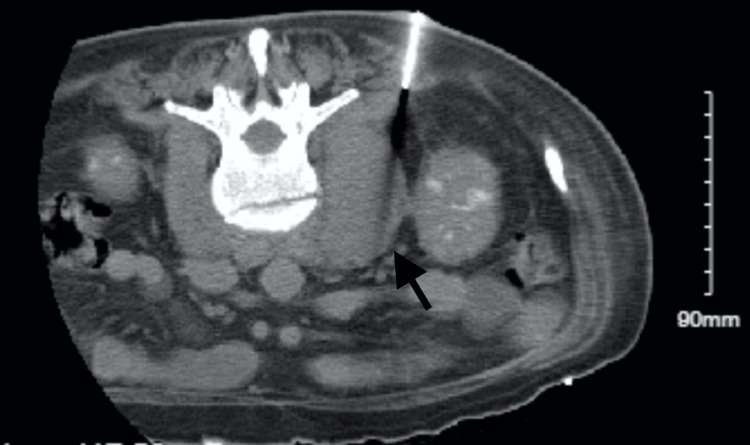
CT scan with axial view showing percutaneous drainage of left psoas abscess
